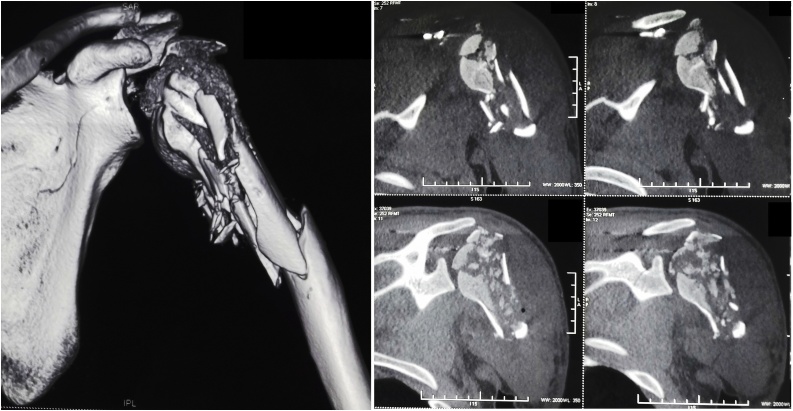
CT scan showing the comminuted fracture of the upper extremity of the humerus.
